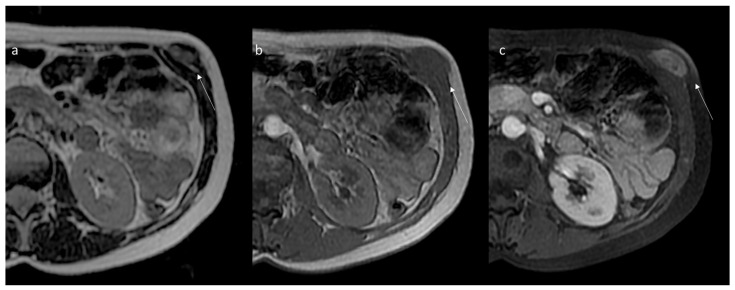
Desmoid of the anterior abdominal wall. MRI axial slices in T2 (a), T1 (b), and gadolinium-enhanced T1 (c) sequences. A nodular lesion is evident within the muscular and fascial planes of the internal and external oblique muscles (arrow).
